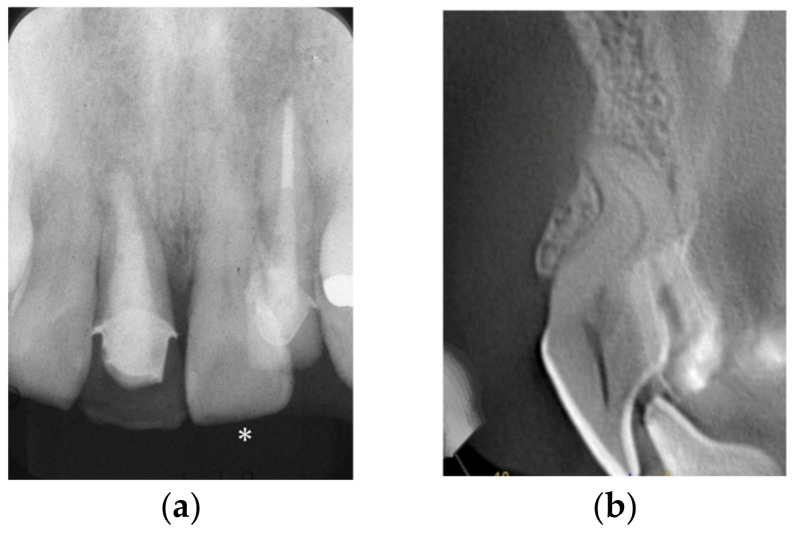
Comparison of diagnostic imaging for a maxillary left central incisor (∗). Periapical radiograph (a) and corresponding preoperative CBCT scans (b). The three-dimensional imaging reveals the intricate internal anatomy and provides critical information for establishing a safe path to the apex.
